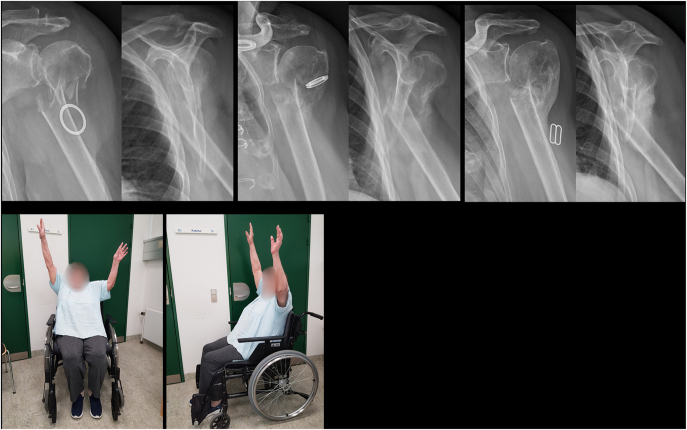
A 79-year-old female with a severely displaced surgical neck fracture. There is no bony contact on the lateral view and limited contact on the anterior–posterior view. The fracture healed with a lateral and posterior bone bridge. Radiographs were taken at admission, 6 weeks, and 6 months. Photographs were taken at 6 months.
